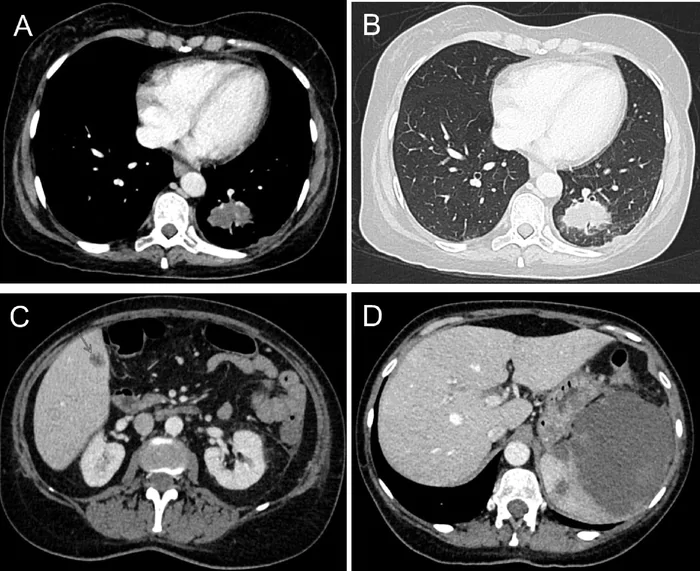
Axial contrast-enhanced computed tomography (CT) scans show a consolidative lesion in the left lower lobe on (A) mediastinal and (B) lung window settings. (C) A slightly hypodense lesion is identified in hepatic segment V (arrow). (D) A hypodense splenic lesion is observed in the same location as on the CT scan performed 2 years earlier (Figure 1B), although with an increase in size.
